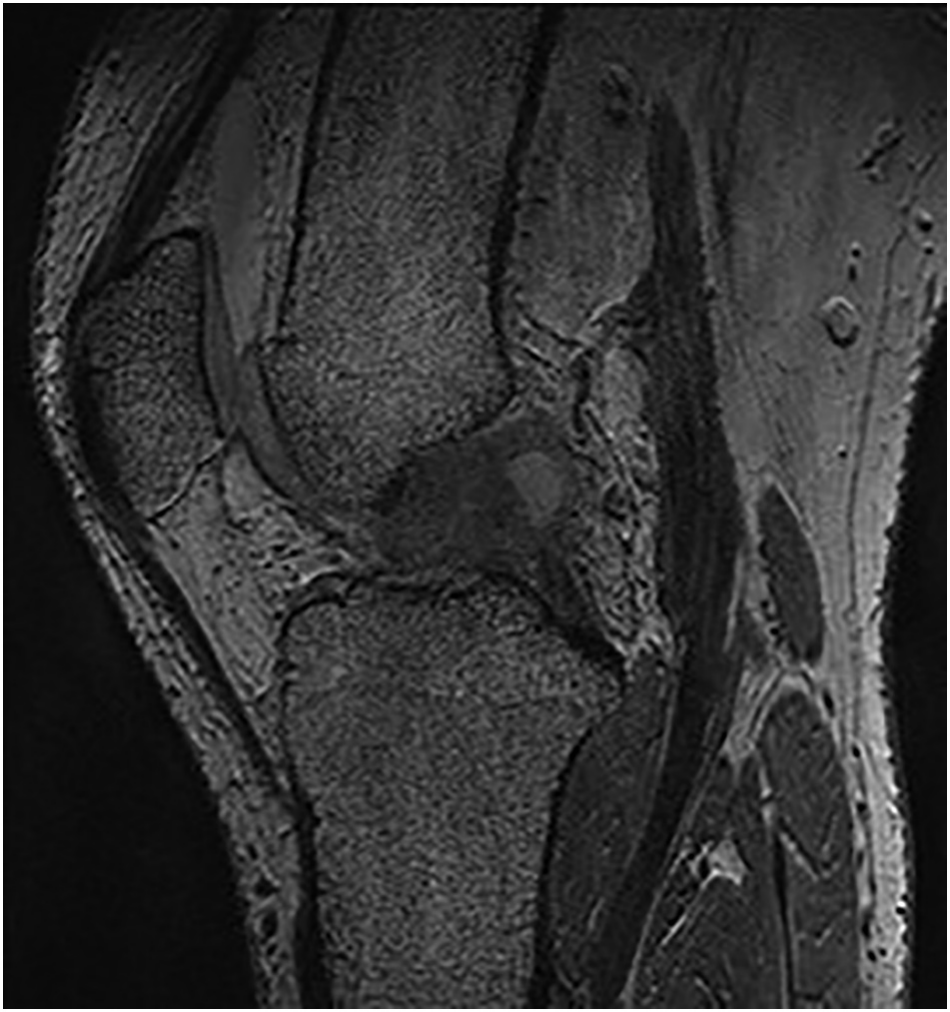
Sagittal T3 MRI image (gradient echo) again revealing a mass within the notch of the knee. Minimal blooming of the lesion is appreciated.
